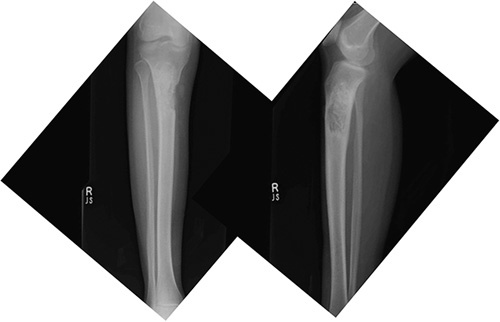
A 19-year-old girls presented with pain in right knee after a blunt trauma. Underwent aspiration and cytology showed no malignant cells. Eventually misdiagnosed as GCT. X-ray tibia anteroposterior and lateral views showing a lytic lesion at proximal tibia in which curettage and bone grafting was done. Final pathologic report is osteogenic sarcoma. Presented to our institute with recurrence/residual tumor. GCT indicates giant cell tumor.
